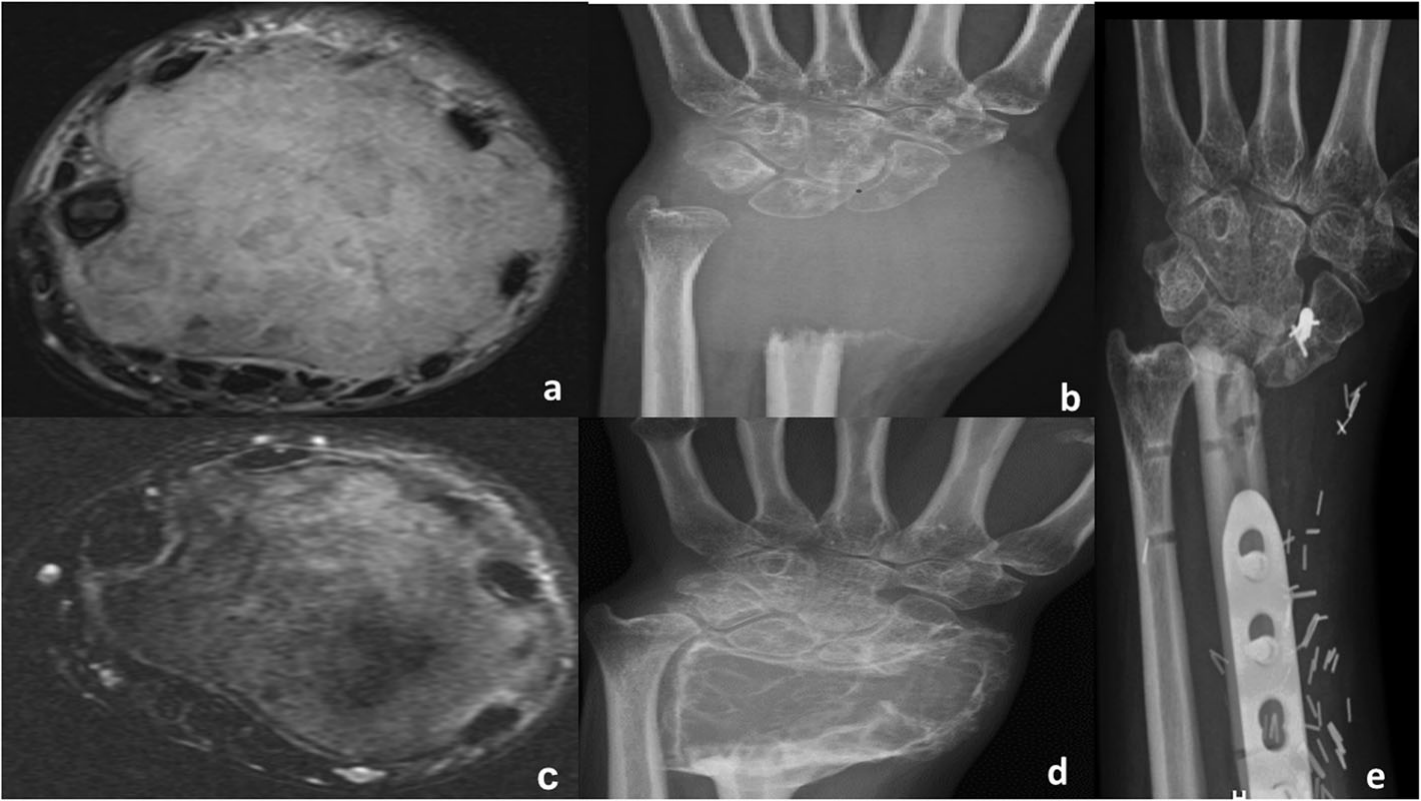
A 30-year-old female with giant cell tumor of distal radius treated with resection. MRI (a) and AP radiograph (b) before treatment show locally aggressive lytic lesion with ambiguous borders and after treatment (c, d) reveal internal mineralization of the lytic areas and clear sclerotic margin. Postoperative AP radiograph (e)
